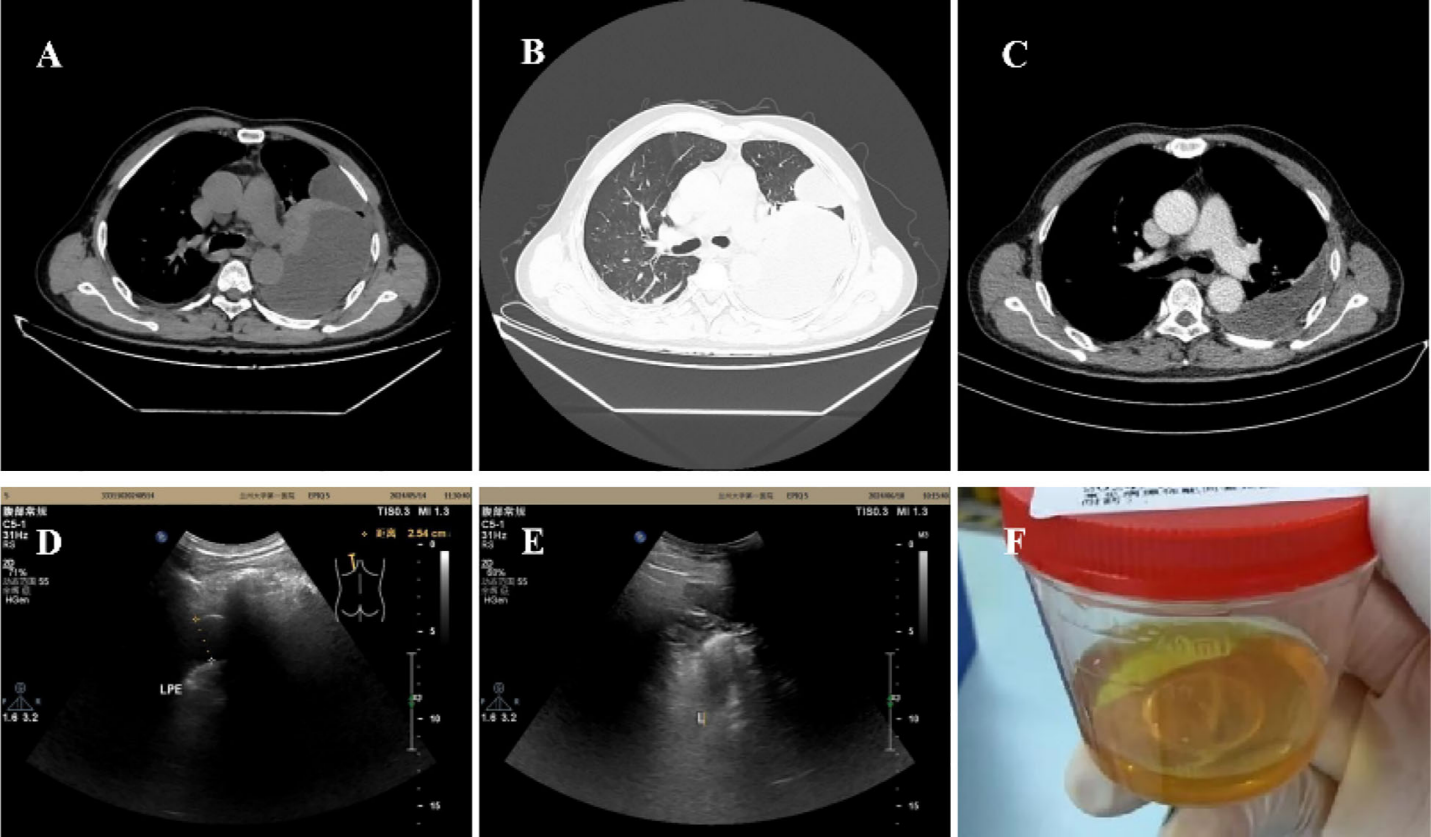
(A, B) On admission, CT showed a left-sided pleural effusion, partially encapsulated. (C) CT showed pleural effusion was signaficatlly decreased 15 days after treatment. (D) 30 days after discharge, ultrasound showed the left thoracic cavity (posterior axillary line, scapula level) had 10 mm and 25 mm pleural effusion, respectively. (E) Pleural effusion was completely absorbed 66days after discharge. (F) Jelly-like pleural fluid was withdrawn on admission.
